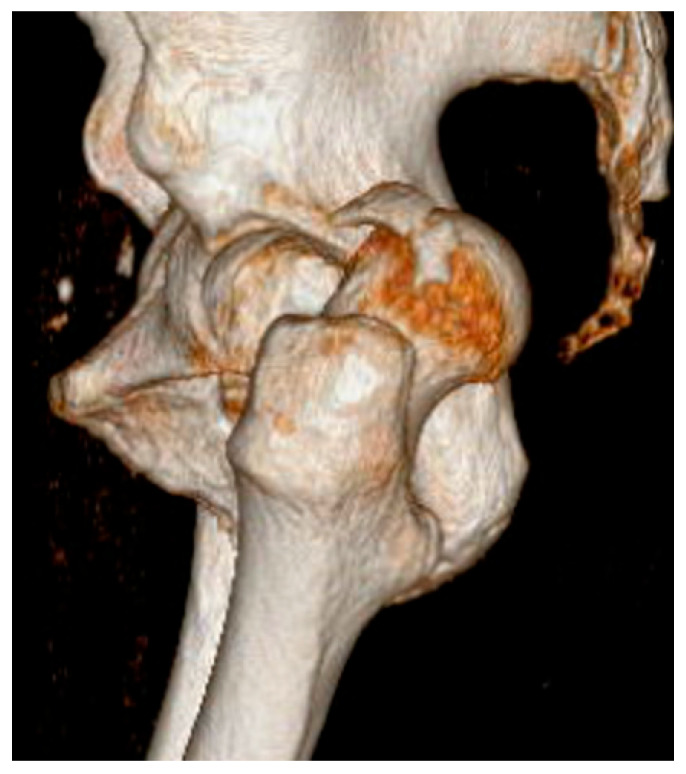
3D reconstruction of the CT of the Pipkin 4 fracture with hip dislocation.
